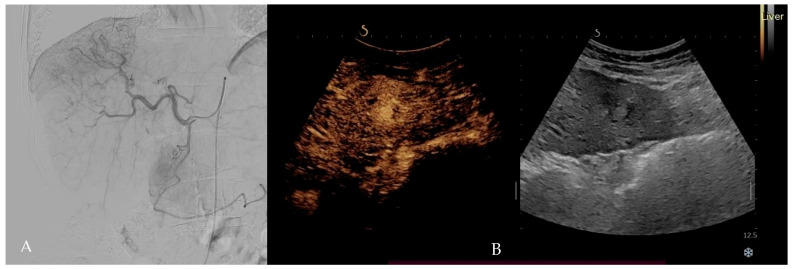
IA-CEUS—intra-arterial contrast-enhanced ultrasound of an HCC nodule of VII liver segment. (A): Angiographic phase; (B): arterial phase (20 secs) of IA-CEUS.
